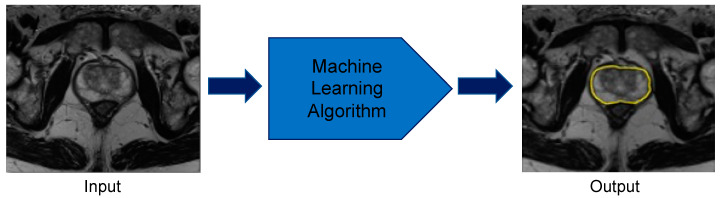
Prostate organ segmentation performed by machine learning methods. The computer takes multiparametric magnetic resonance imaging images as inputs and applies the developed machine learning algorithm to correctly identify the borders of the prostate.
